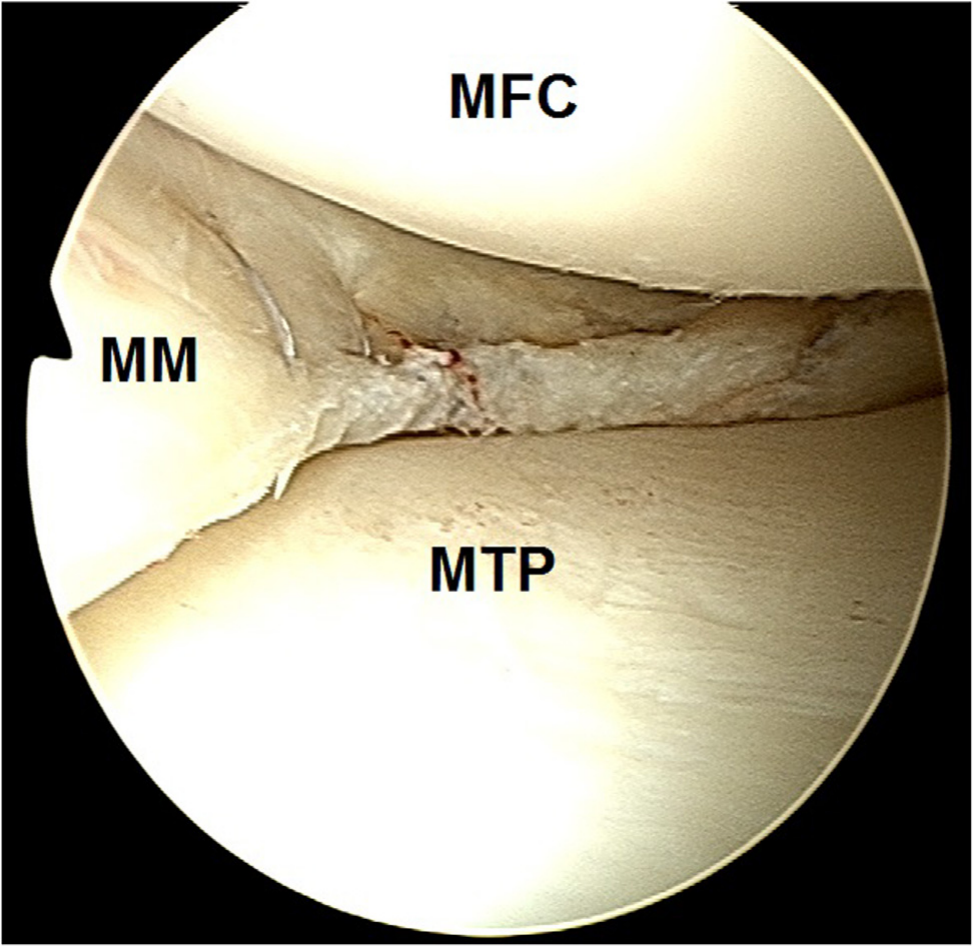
Arthroscopic view of a left knee using a 30° arthroscope (Olympus Inc) through the anterolateral portal. The medial meniscus bucket handle fragment is reduced to the anatomic location and repaired with multiple inside-out sutures as described previously.10.Thigh tourniquet cuff (Zimmer) is now inflated to a pressure of 250 mm Hg.11.Full-thickness ipsilateral quadriceps tendon harvesting begins, measuring 9 to 10 mm wide and 80 to 90 mm long. Harvest is initiated proximally and the tendinous part of the graft is whipstitched with a No. 5 Ethibond suture (Ethicon). A 15- to 18-mm-long proximal patella bone plug, in accordance with the preoperative CT planning, by 9 to 10 mm wide is measured and harvested, ensuring to leave an 8- to 10-mm-wide bone bridge between the persistent distal patellar bone deficiency site and the current proximal patellar bone plug harvest site (Fig 20). The tendinous part is lifted throughout bone plug harvest to identify and protect the deep attachment area of the quadriceps tendon to the patellar bone as a safety measure to avoid inadvertent violation of the deep tendon–bone insertion during bone plug harvest. The quadriceps harvest site is irrigated, and soft tissue layers are closed.
